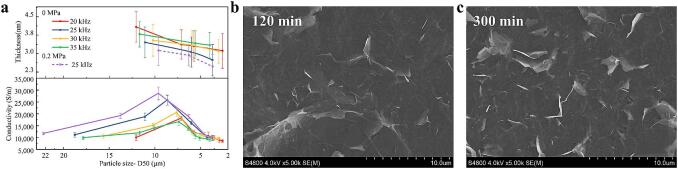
a: Afm characterization results and conductivity changes of flg prepared at different frequencies. b and c: FLG-CMC films prepared by 25 kHz dumbbell probe ultrasonic 120 min and 300 min at 0 MPa.
